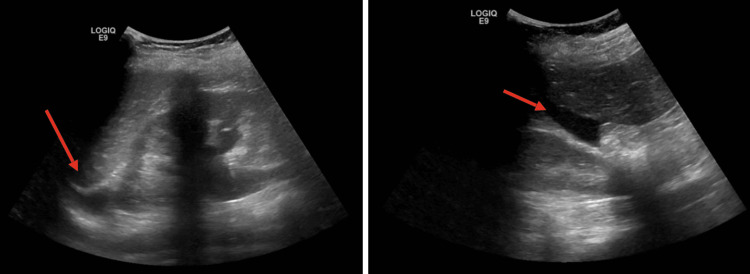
Doppler ultrasound of the upper abdomen shows hyperechogenic nodular images with imprecise limits and the presence of free fluid in the hepatorenal window
